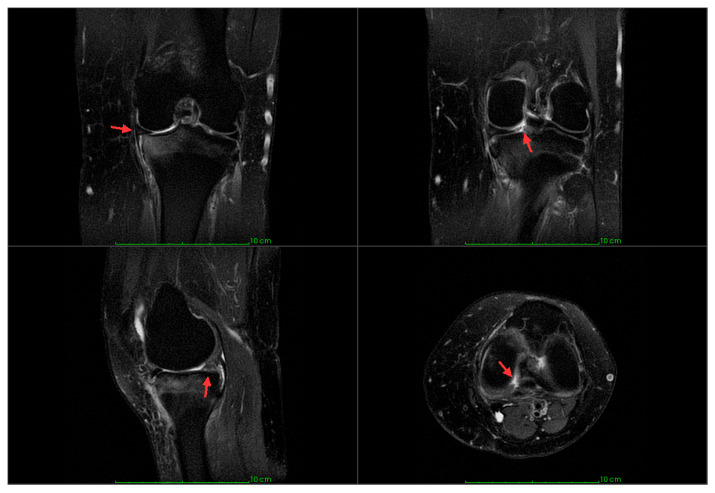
MRI findings of MMPRT. (Upper left) Coronal proton density-weighted fat-saturated (PD-FS) image showing meniscal extrusion (red arrow) and subchondral edema of the tibia. (Upper right) Coronal PD-FS image demonstrating the cleft sign (red arrow) (Lower left) Sagittal T2-weighted fat-saturated image with the ghost sign (red arrow). (Lower right) Axial PD-FS image with a radial tear at the root (red arrow).
